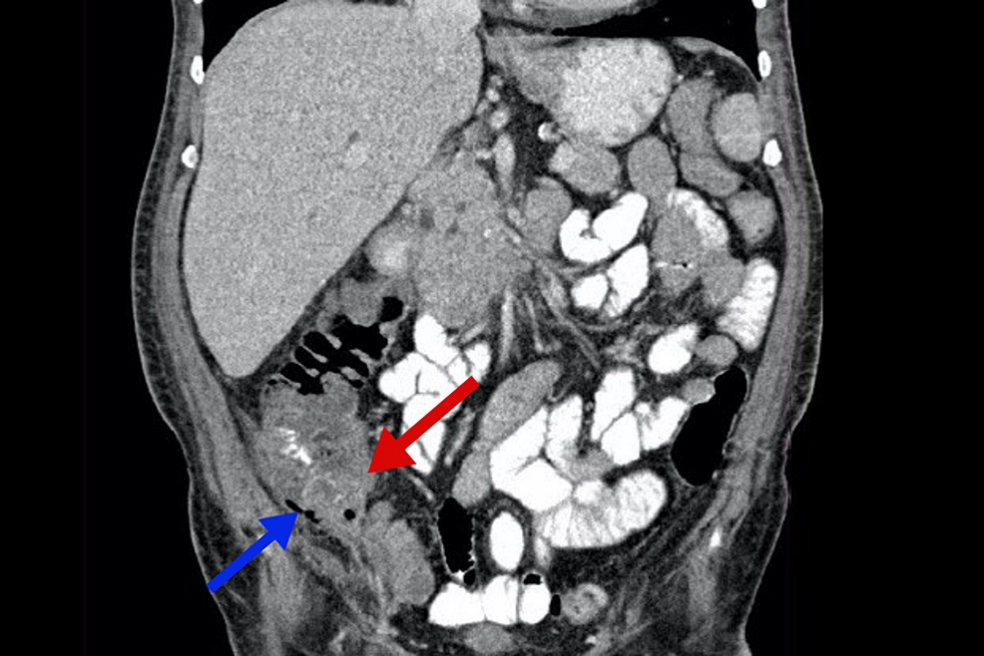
Coronal oral contrast CT scan of the abdomen and pelvis showing pericecal collection of fluids and gases, which is suggestive of leakage The red arrow points to the pericecal fluid collection, while the blue indicated the gas collection.
